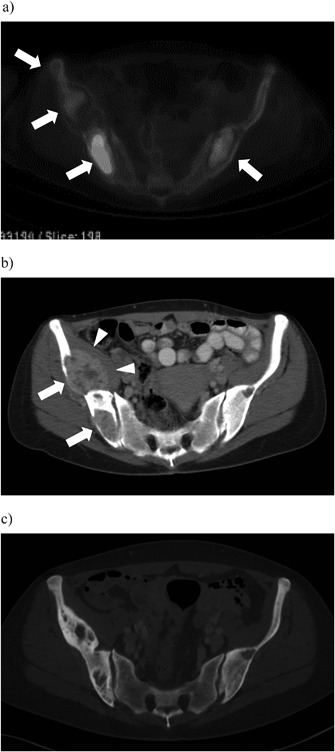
(*A*) ^18F^FDG PET/CT shows marked uptake in the lytic lesions of both iliac bones indicating high metabolic activity (arrows). January 9, 2007. (*B*) Contrast‐enhanced CT demonstrates lytic lesions within both iliac bones (arrows) with a large metastatic soft tissue mass (arrowheads) dislocating the iliacus muscle. November 30, 2006. (*C*) Contrast‐enhanced CT 10 years after successful treatment shows ossification of the old lytic lesions with no signs of aggressive expansion. March 9, 2017.
